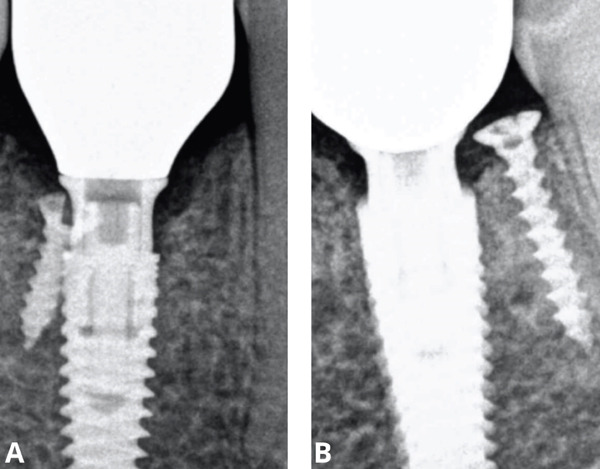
Radiographic evaluation: (A) Implant 36. (B) Implant 46.
